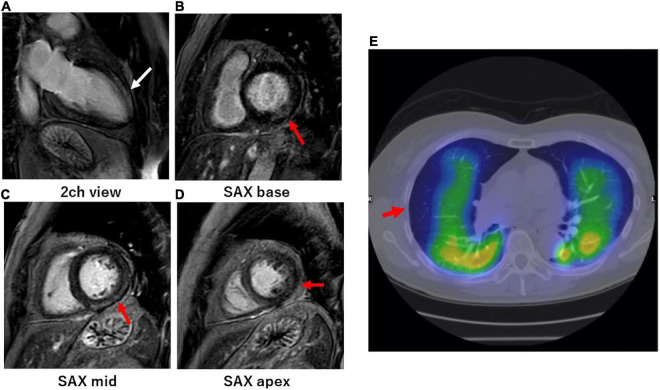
Cardiac magnetic resonance of myocardial injury and SPECT/CT of pulmonary embolisms in representative long COVID patients. (A–D) A woman in her 60s visited the cardiology outpatient clinic because of persistent dyspnea and palpitations. The patient was severely ill during the acute phase of a COVID-19 infection and was temporarily managed with a ventilator. She underwent CMR at the outpatient clinic 1 month after the onset of persistent dyspnea and palpitations. CMR-LGE imaging in the 2-chamber view (A) showed intramyocardial enhancement in the mid-distal anterior wall of the left ventricle (white arrows). CMR-LGE imaging in the short-axis views (B–D) also showed endomyocardial (B) and subepicardial (C,D) enhancement on the basal-distal inferolateral wall, respectively (red arrows). (E) A woman in her 60s visited the cardiology outpatient clinic because of persistent dyspnea and chest pain. The patient was mildly ill during the acute phase of the COVID-19 infection. Lung perfusion SPECT/CT at the outpatient clinic revealed defects in the middle and lower peripheral lesions in the right lung (red arrows). Her COVID symptoms persisted for more than 6 months.
